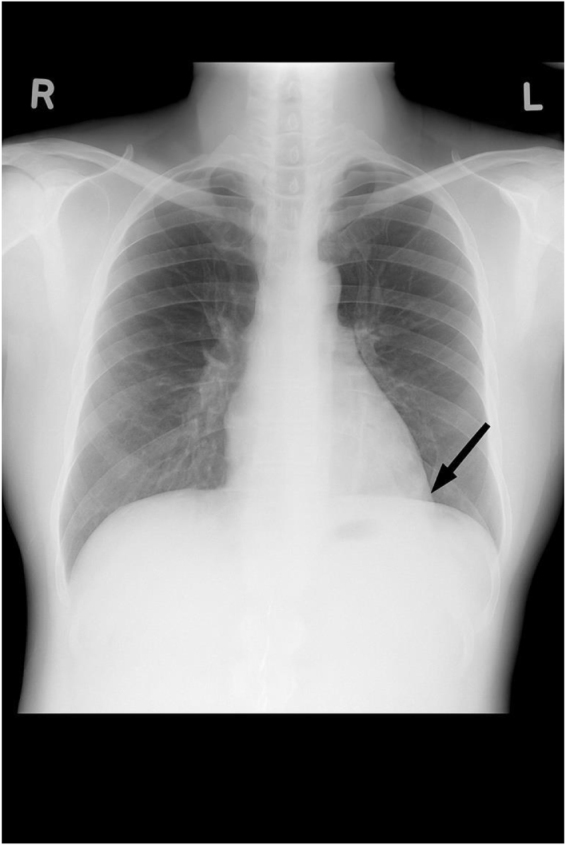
X-ray shows blunting of the right costophrenic angle but no parenchymal infiltrates or cavitary lesions were present.
